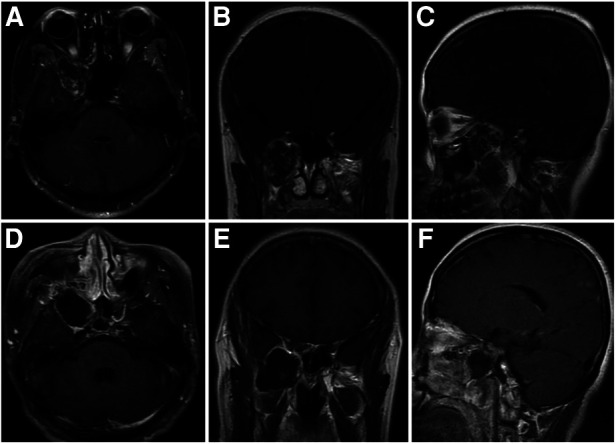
Case 1: a 46-year-old woman with a schwannoma in the PPF. A preoperative T1-weighted enhanced MRI (A–C) shows the tumor located in the PPF. A postoperative T1-weighted enhanced MRI confirms the total removal of the tumor by the PRLMA (D–F).
